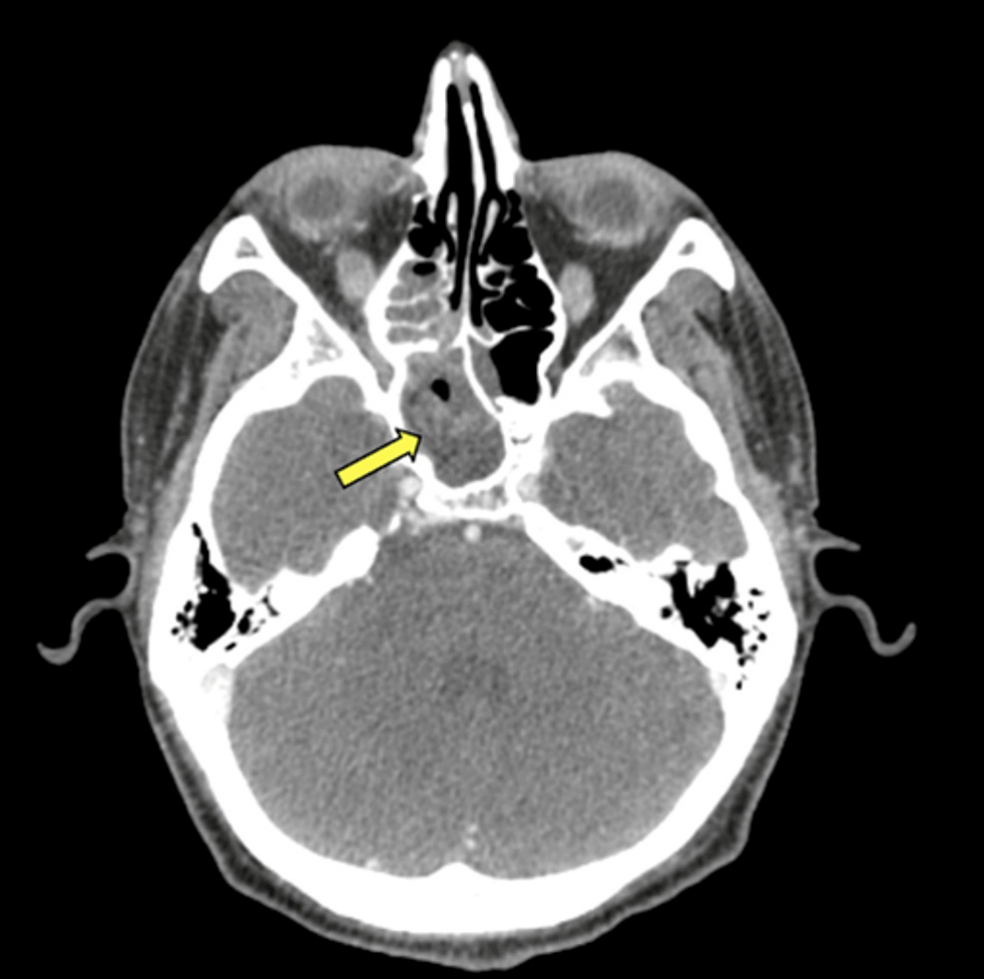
Initial CT maxillofacial with contrast Findings concerning for invasive fungal sinusitis with near complete opacification of the right sphenoid sinus (yellow arrow).
